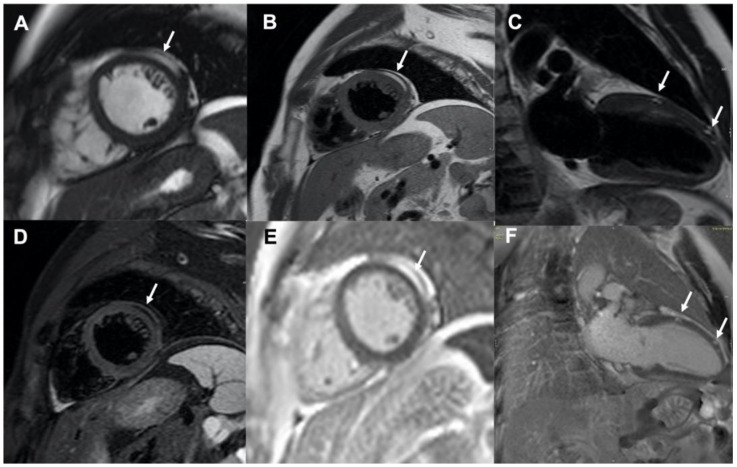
Cardiac magnetic resonance imaging showing multiple areas of intramyocardial fat deposition (white arrows); (panel A): b-steady-state free precession cine sequence, mid short-axis view; (panels B,C): T1-weighted black-blood Turbo Spin Echo images, mid short-axis view and 2-chamber view, respectively; (panel D): T1-weighted black-blood Turbo Spin Echo image with fat suppression, mid short-axis view; (panels E,F): late gadolinium enhancement images, mid short-axis view and 2-chamber view, respectively.
